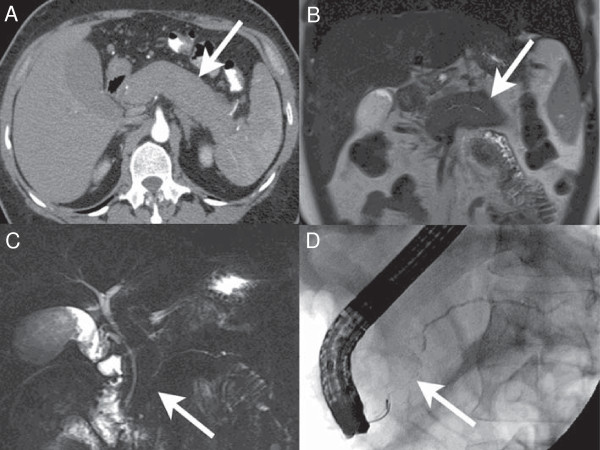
Diffuse autoimmune pancreatitis. A. Axial CT image in the pancreatic parenchymal phase shows the typical enlarged, poorly enhancing gland (arrow). Note the lack of inflammatory change around the organ which differentiates the disease from acute pancreatitis with necrosis. B. Coronal T2 Weighted MR image demonstrates low signal intensity in the pancreas (arrow) due to the diffuse fibrosis in the gland. C. Coronal MRCP image depicts a diffusely irregular pancreatic duct with stenosis distally in the pancreatic head (arrow). D. ERCP confirms the MR findings including the ductal stenosis (arrow).
